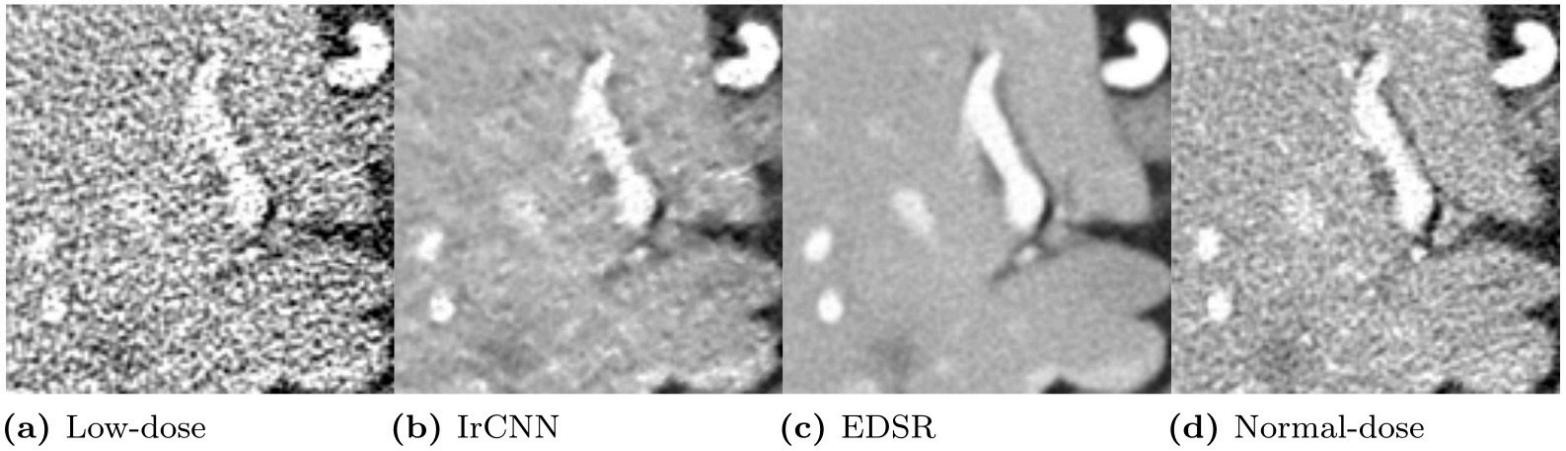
Close-up examples of (a) the original low-dose computed tomography (CT) image, (d) the normal-dose reference image, and noise-reduced CT image results from two different networks, (b) IrCNN (PSNR: 37.408) and (c) EDSR (PSNR: 39.875).
